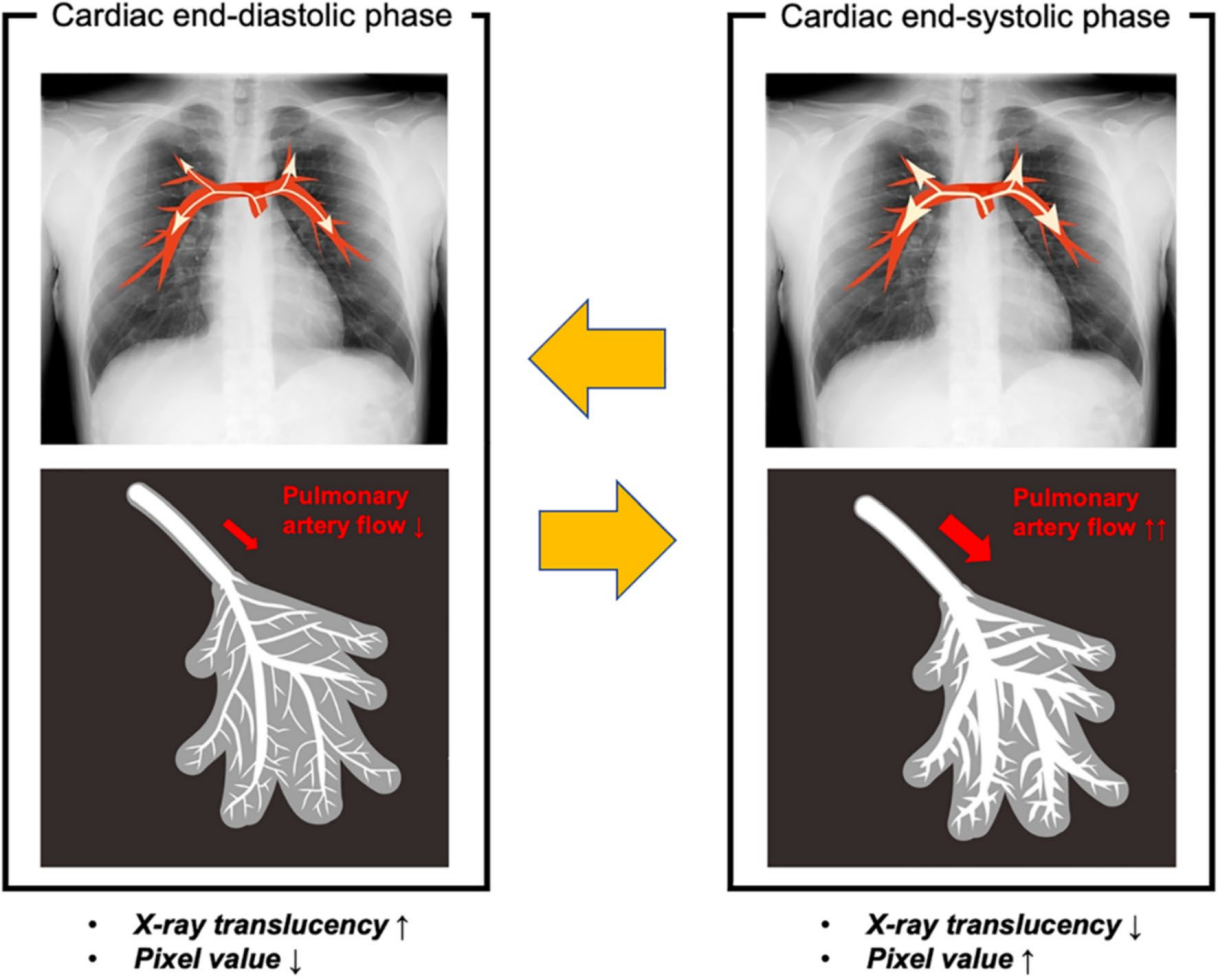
Theory of perfusion imaging of dynamic chest radiography. Pulmonary artery flow increases during the systolic phase, leading to increased blood and vessel volume in the lungs. This results in a slight temporal decrease in X-ray translucency. Dynamic chest radiography analyzes small temporal changes in X-ray translucency (pixel value) by cardiac pumping and visualizes the pulmonary perfusion. * This figure was adapted from Ref. 60 under the Creative Commons Attribution 4.0 International License
